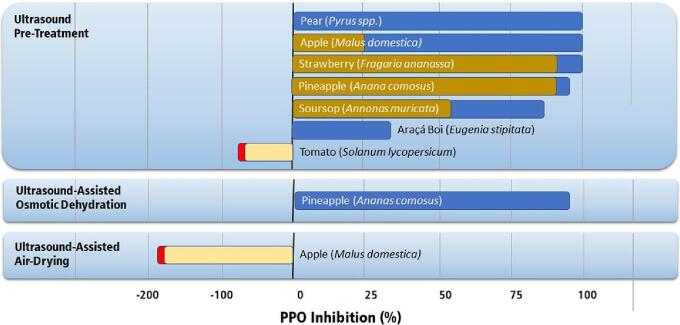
Highest inhibition of PPO in fruits by applying ultrasound technologies [25], [99], [156], [157], [160], [161], [162], [163], [164]. Single-colored bars indicate unique values in the literature. Double-colored bars indicate the lowest (brown and yellow) and highest (blue and red) values reported in the literature.
